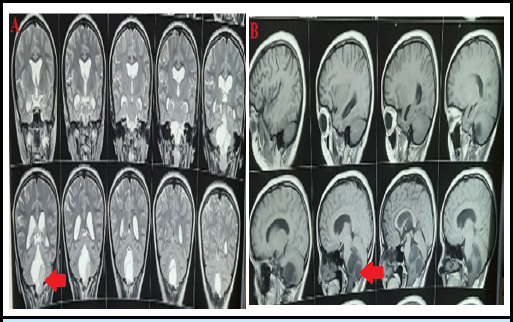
MRI showing posterior fossa tumor extending to the spinal cord in both T2 coronal view (pane A) and T1 sagittal view (pane B).
